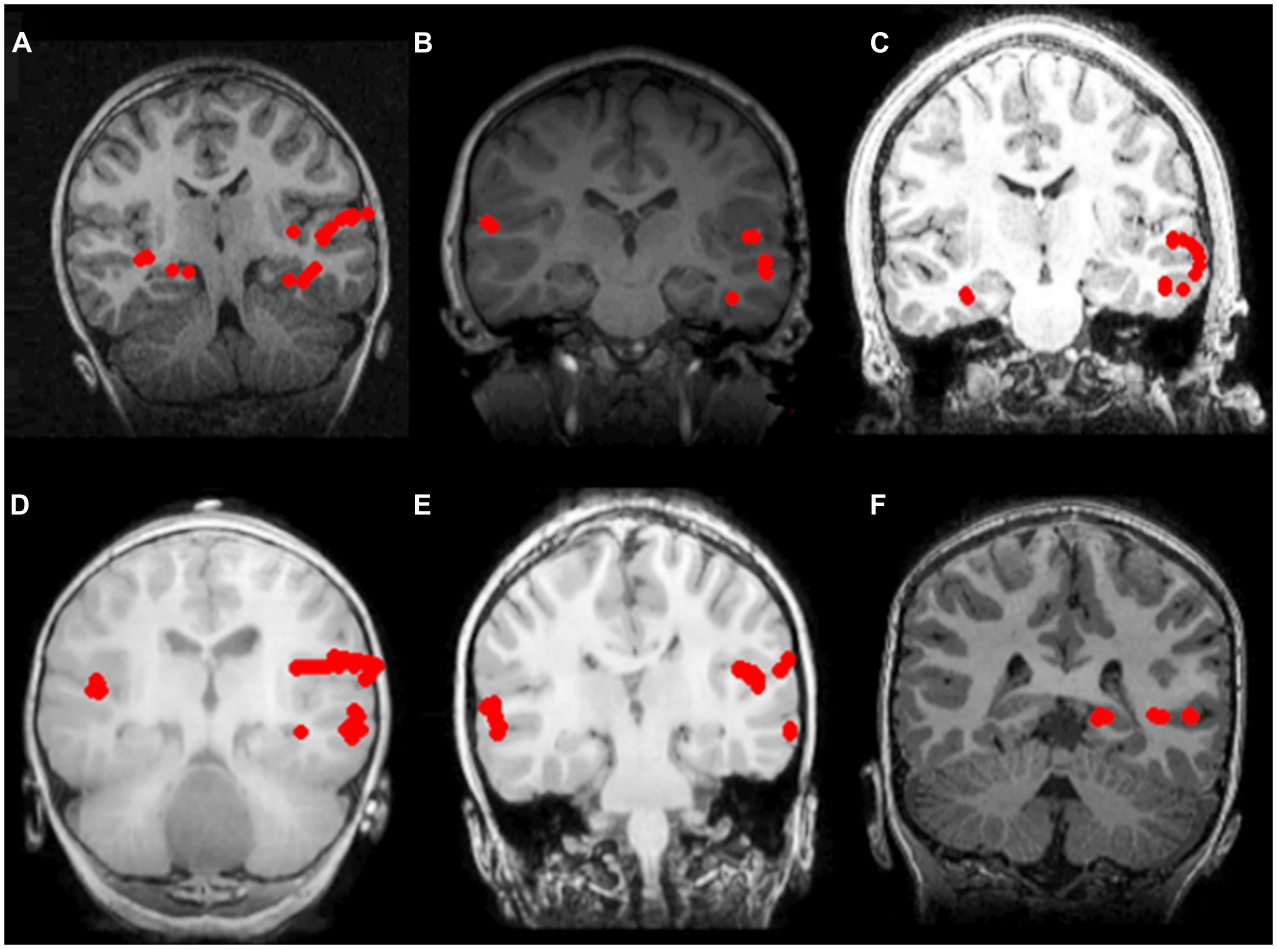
Receptive language mapping with MEG with and without sedation. Language activity (dipolar) sources are represented as solid red circles projected onto the patients’ MRI, displayed in radiological convention. Left hemispheric dominance for receptive language in patients evaluated without sedation (A–C), and with sedation (D–F). (A) 7 year-old female with symptomatic frontal lobe epilepsy; (B) 8 year-old female with ganglioglioma of the left temporal lobe; (C) 16 year-old male with symptomatic partial seizures of right temporal lobe origin; (D) 2 year-old male with cervical medullary tumor; (E) 6 year-old male with symptomatic epileptic spasms of right hemisphere origin; (F) 6 year-old male with symptomatic partial seizures of left temporal lobe origin.
